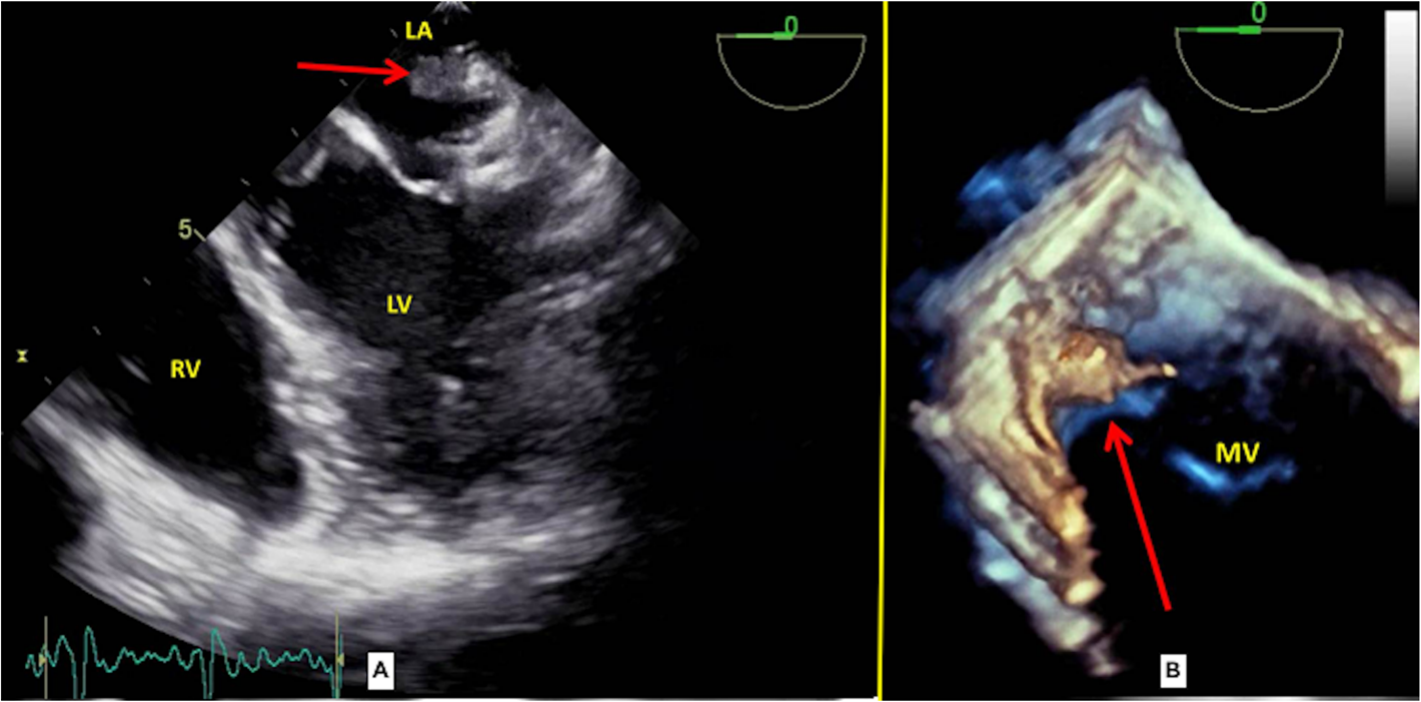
Intra-operative trans-oesophageal echocardiography—A vegetation in the left atrium (red arrow) away from the mitral valve and B 3D reconstructed image of the same showing the vegetation (red arrow)
